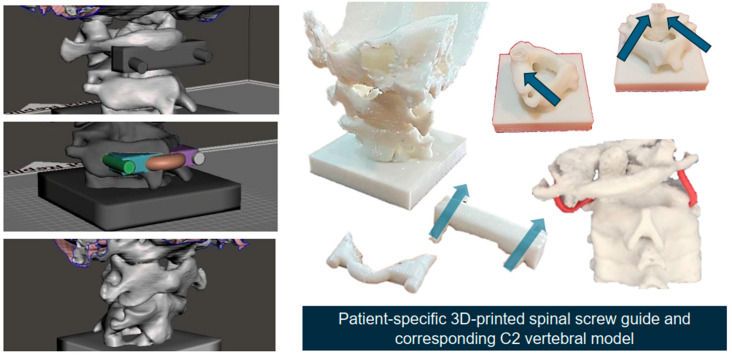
The 3D-printed models enhance the surgeons’ ability to visualize and assess anatomical variations, practice surgical techniques, and plan patient-specific procedures, contributing to improved surgical precision and patient outcomes. The patient-specific, 3D-printed spinal screw guides and corresponding C2 vertebral models are derived from CT imaging data. It highlights advancements in personalized spine surgery planning and execution. The dark blue arrows indicate the trajectory of the screws, while the light blue arrows represent the direction of the screw guides.
